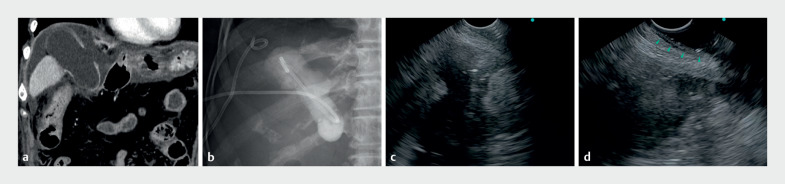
Gel immersion technique in endoscopic ultrasound-guided gallbladder drainage (EUS-GBD).
